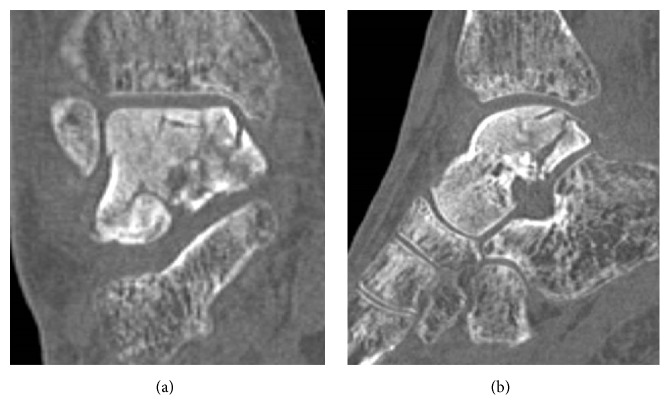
Preoperative CT showing sclerotic change and collapse of the talar body in coronal (a) and sagittal (b) views.
